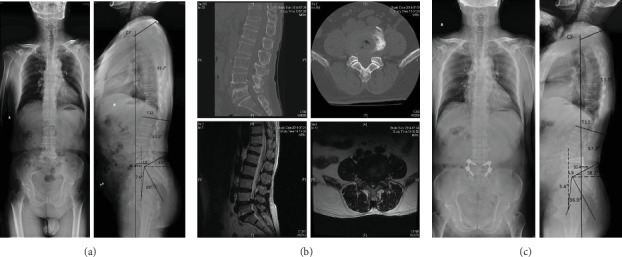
A 67-year-old male with diagnosed with lumbar spinal stenosis and had back pain for 3 years, with intermittent claudication. (a) Preoperative X-ray showed SVA = 46.3 mm, SS = 32, Roussouly type II, lumbar lordosis apex at L4 base, GL = 42.1°, PI = 39.0°, PT = 7.6°, SS = 32.0°, GK = 32.2°. (b) Preoperative lumbar CT and MRI showed L4/5 segment disc herniation, yellow ligament hypertrophic, dural compression, and spinal canal narrowing. (c) Postoperative X-ray showed that SVA = 10.4 mm, SS = 38.2°, Roussouly type III, the lumbar lordosis vertex was at the L4 midpoint, GL = 57.2°, PI = 5.4°, PT = 36.9°, SS = 38.2°, GK = 53.5°. SS, GL, and GK increased; SVA and PT decreased; PI unchanged. Lordosis vertex moved up to the L4 midpoint, classification from Roussouly types II to III (standard).
